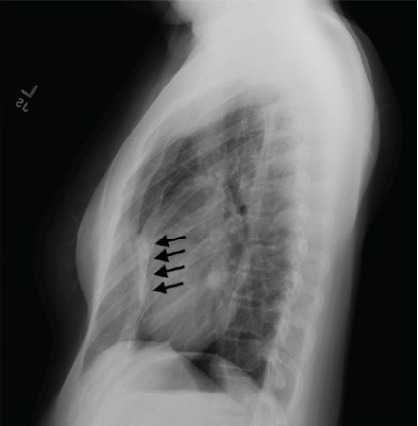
Chest radiograph (lateral view) demonstrating right ventricular compression from severe pectus excavatum (black arrows).
